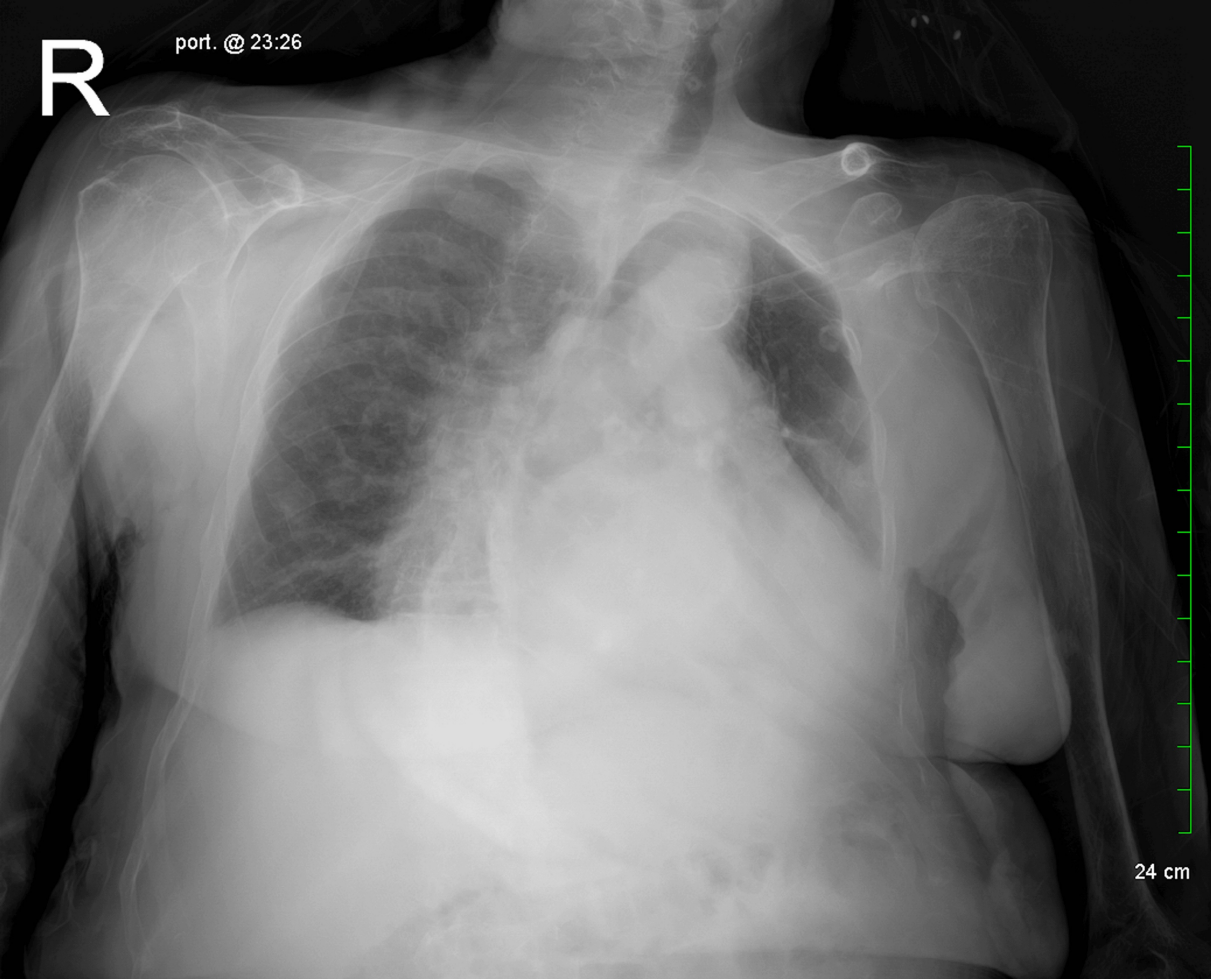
Anteroposterior view X-ray of the chest with the patient rotated to the left. The X-ray shows old fractures, left deviation of the mediastinum, possibly in part due to the resected lung tissue, and cardiomegaly with an unknown density posterior to the heart.
